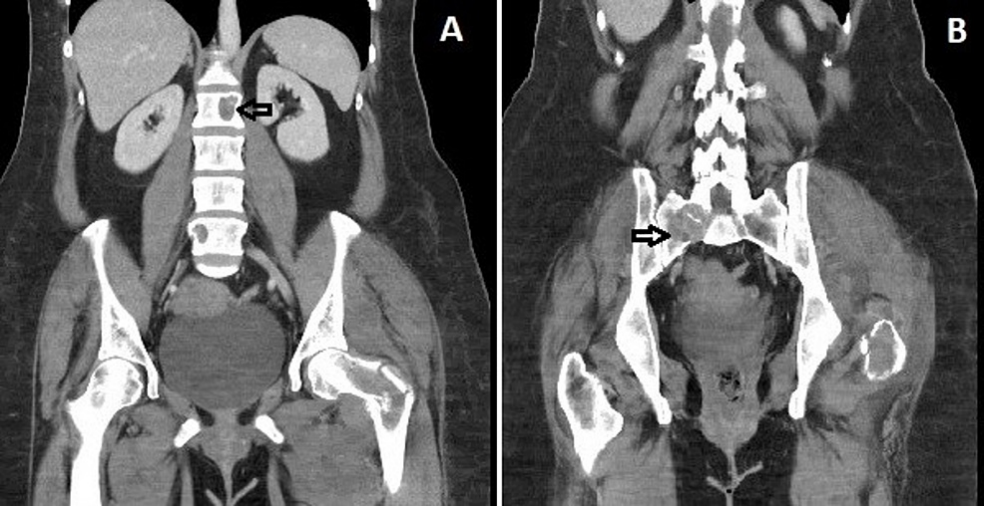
CT scan showing osteolytic bone lesions in (A) lumbar spine (B) ilium.
